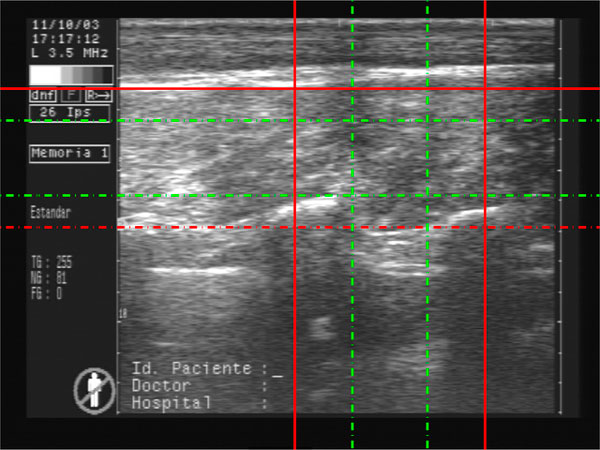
IMF% estimation on ultrasound images. Output image.
